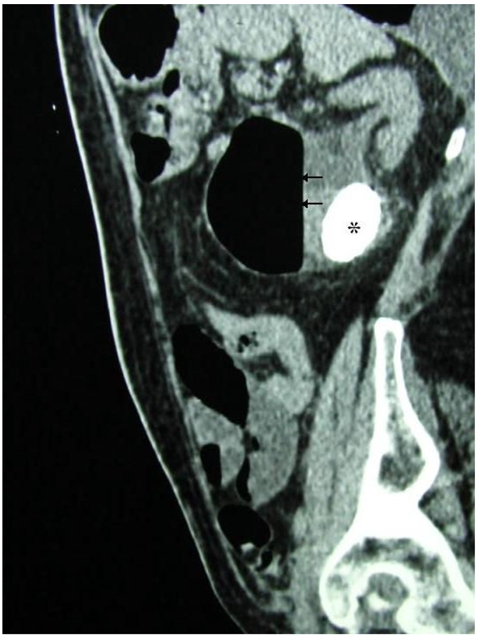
Reformatted sagittal image of contrasted CT scan of the abdomen showing a large obstructing calculus (*) at the left pelvi-ureteric junction, causing gross left hydronephrosis with gas (arrow) noted within the left pelvicalyceal system.
